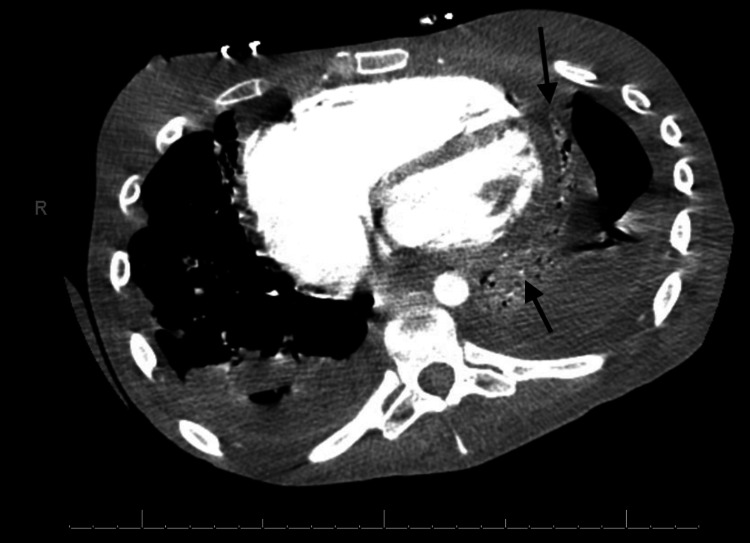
Axial CT chest with contrast showing pericardial effusion (arrows).
